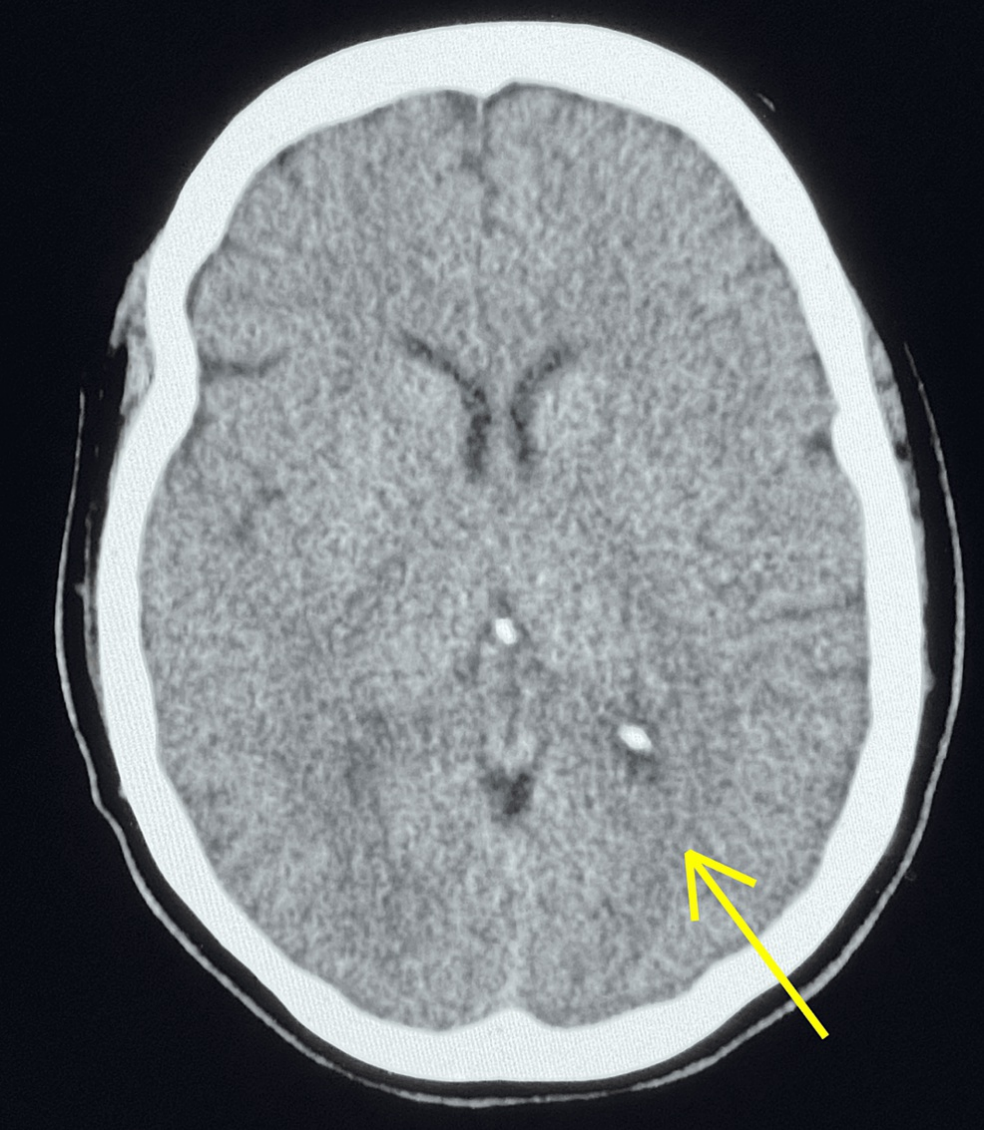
Head CT without contrast, revealing no acute intracranial abnormality CT: computed tomography
